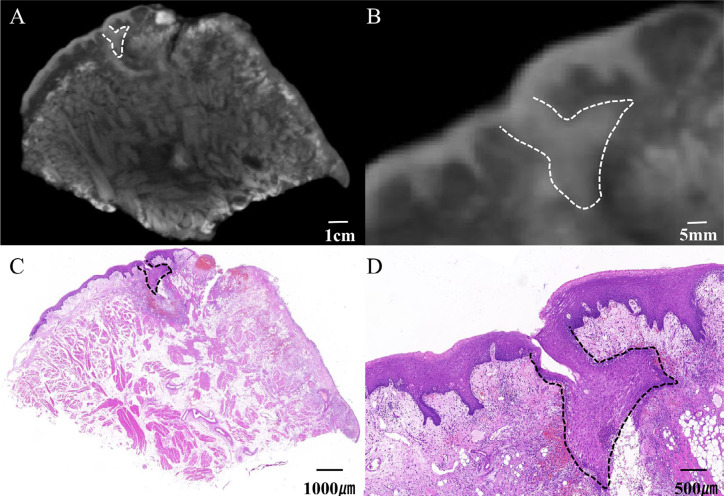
Lugol's iodine-enhanced micro-computed tomography (CT) can identify minimal residual lesions. The dashed line represents the tumor boundary.
